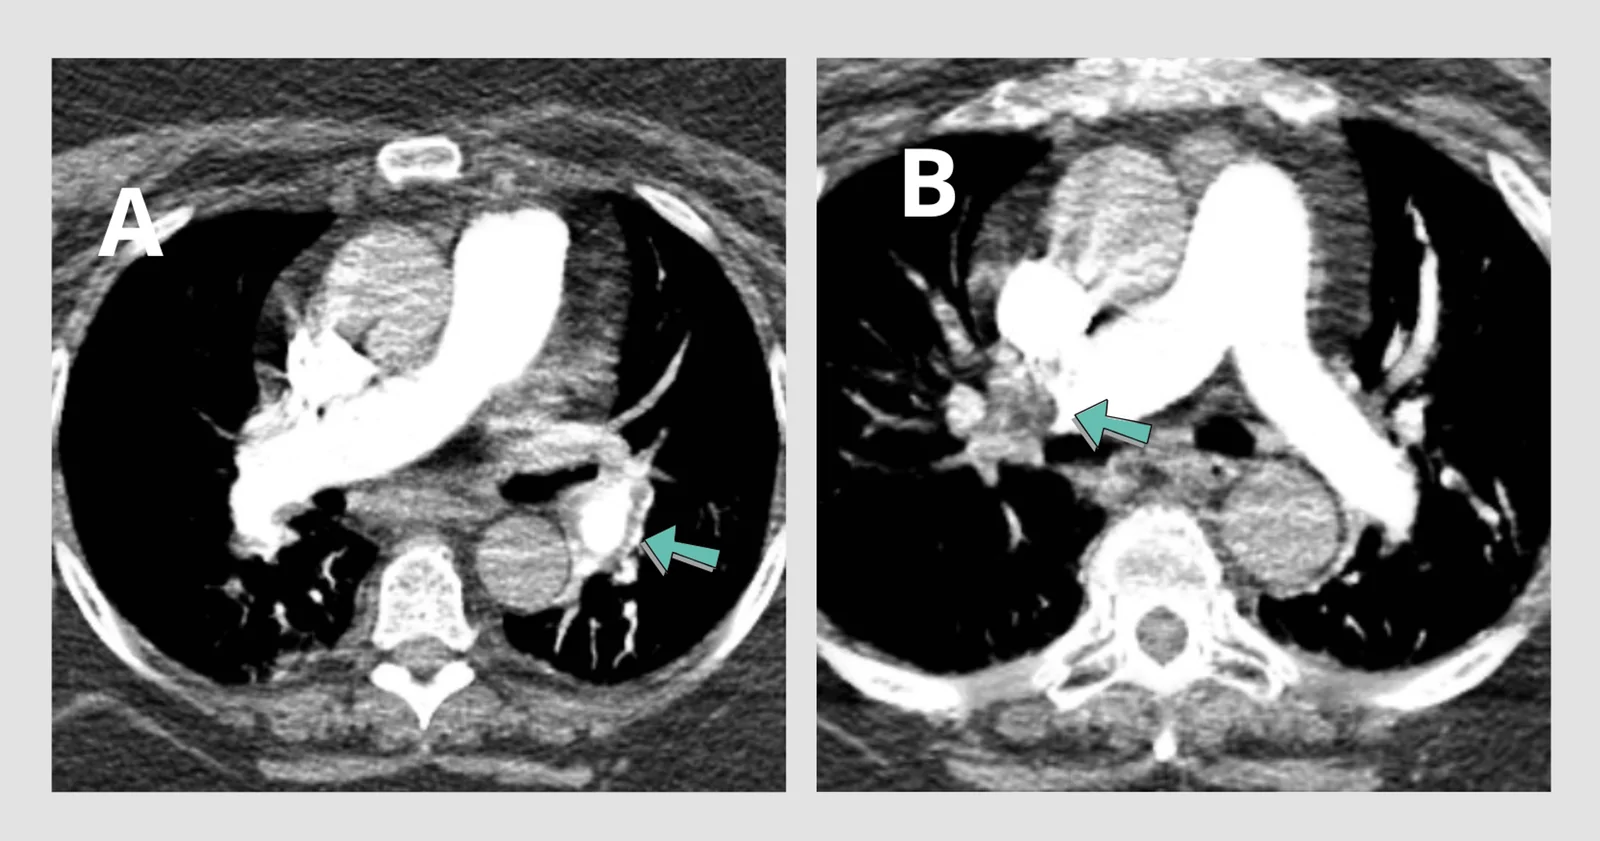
Contrast-enhanced computed tomography pulmonary angiography of the chest performed 24 hours after systemic thrombolysis A and B (axial views): Reconstructions showing a reduction in thrombotic burden within the main pulmonary arterial branches (arrows).
